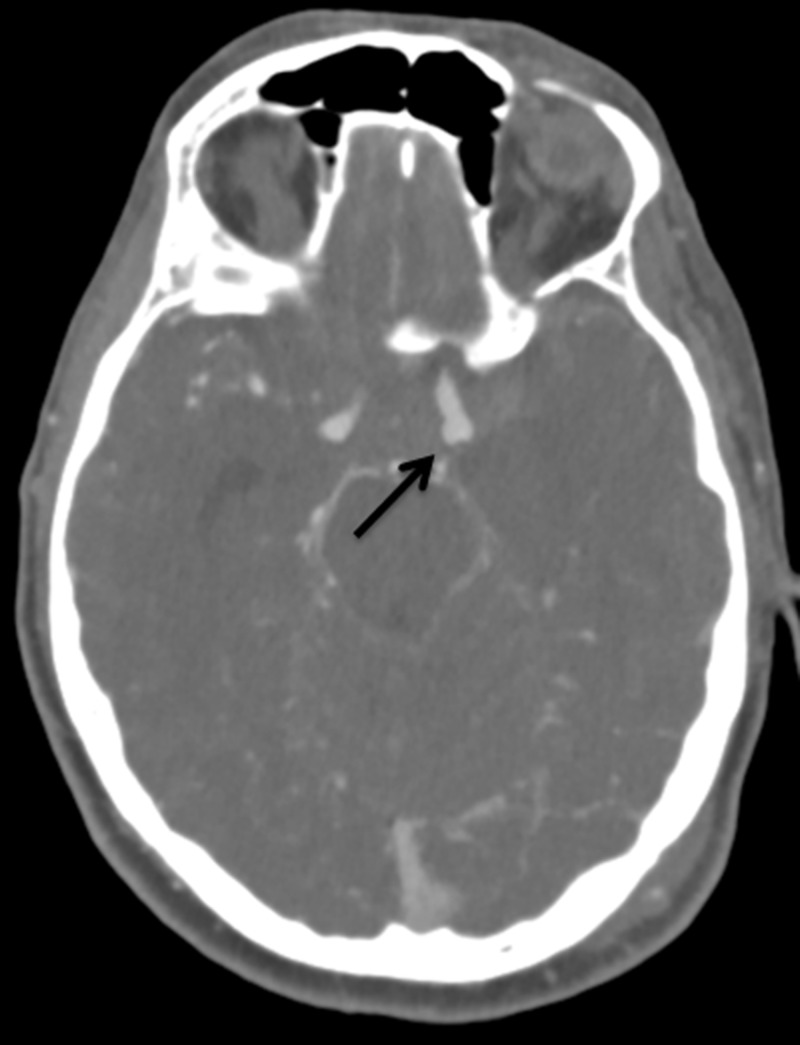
Case 2. Preoperative computed tomography angiography demonstrating the left internal carotid artery dorsal variant aneurysm (arrow).
